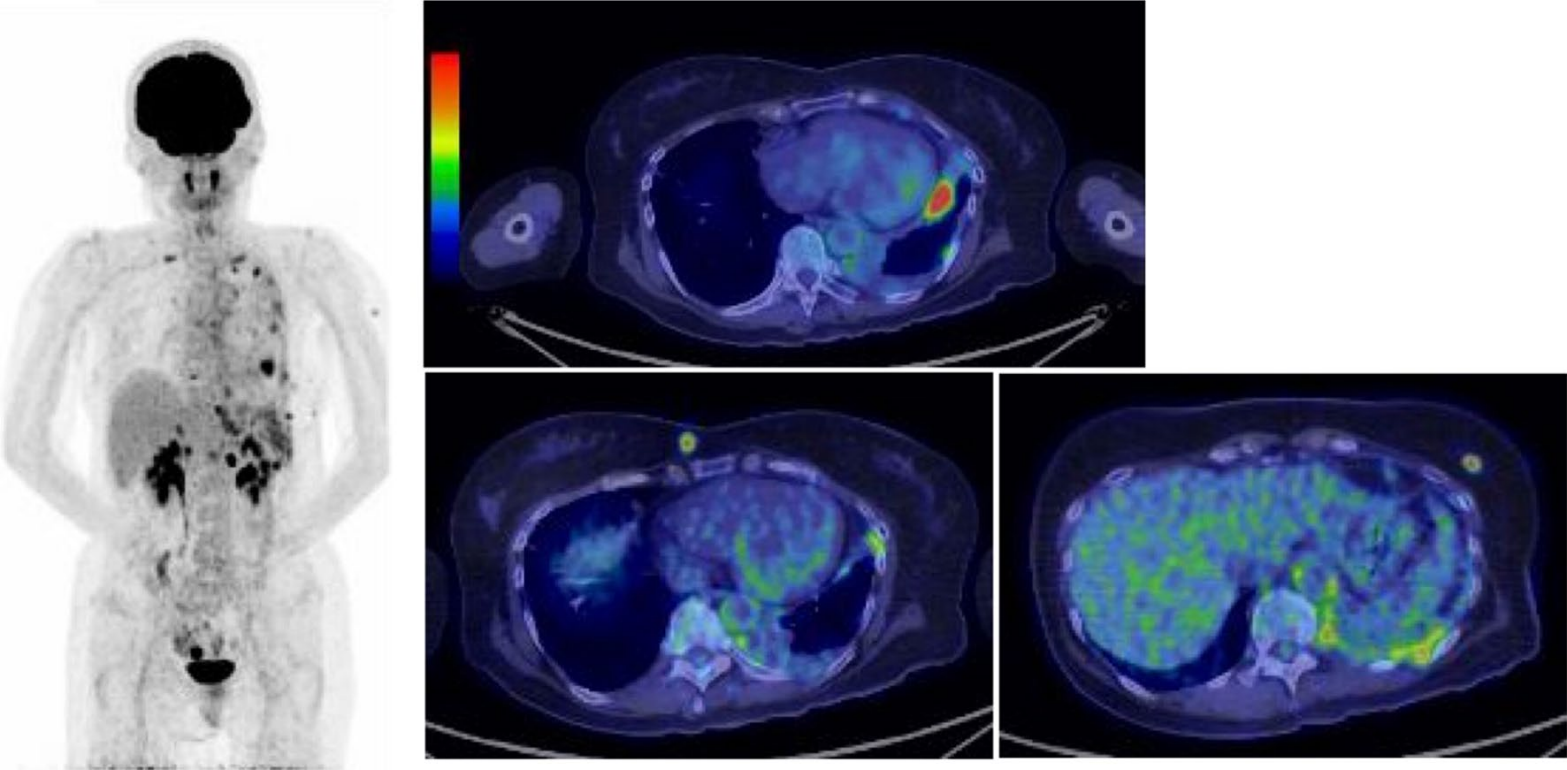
18F-fluorodeoxyglucose (FDG) positron emission tomography-CT showed a nodule with FDG accumulation (SUVmax = 11.42) in the left lower lobe. Multiple FDG accumulations were also observed in the bilateral breasts
